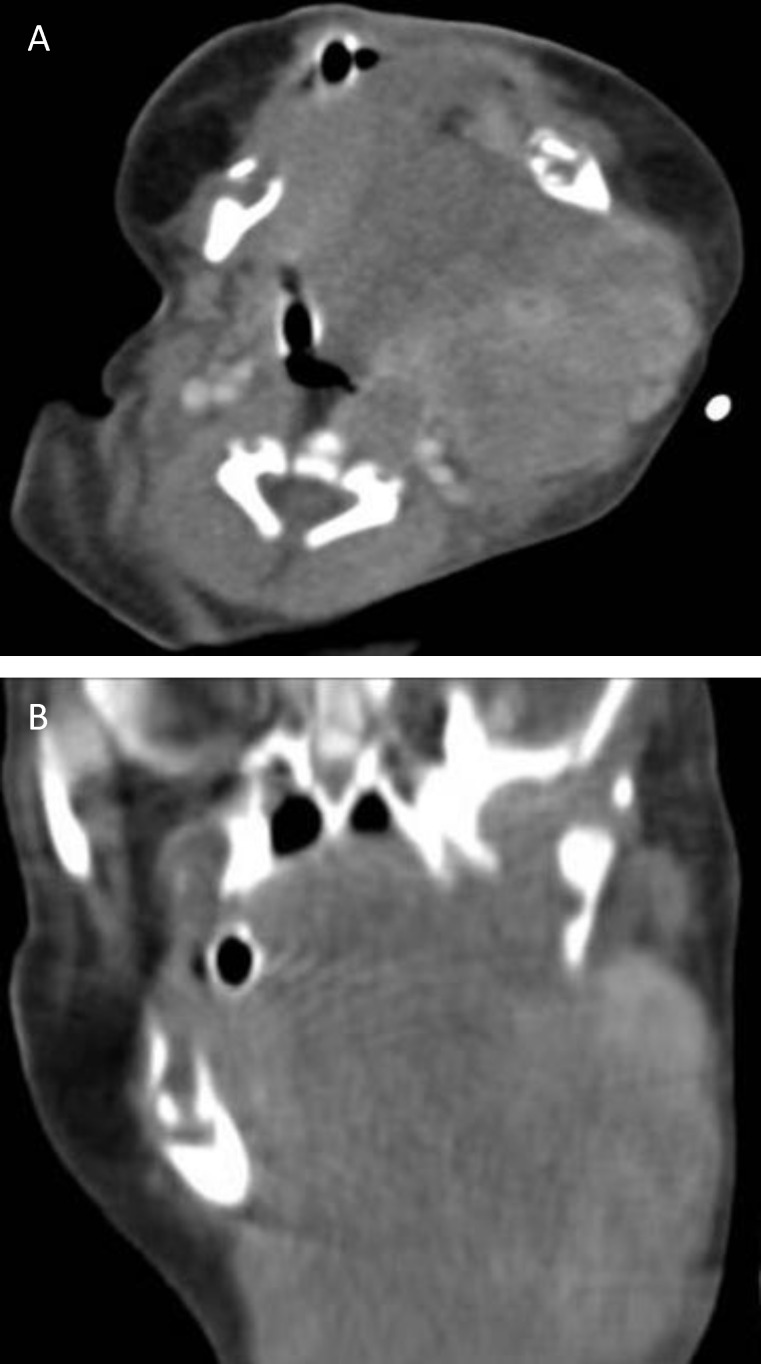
Contrast-enhanced CT in Axial 1,2 mm thickness images (A) and coronal 2mm thickness reconstruction images (B) demonstrate a large multicompartmental  hypodense lesion(arrows) with moderate heterogeneous enhancement in the  left submandibular space involving the sublingual space and oral cavity
